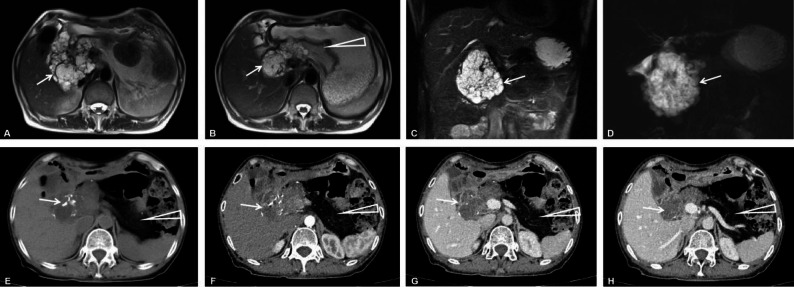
Imaging features. (A, B) Axial magnetic resonance images demonstrate a cystic-solid mass located at the hepatic hilum and pancreatic head, exhibiting a multicystic pattern with visible septations (↑). (C) Coronal magnetic resonance image showing a cystic-solid mass at the hepatic hilum and pancreatic head, also presenting a multicystic pattern with septations (↑). (D) MR cholangiopancreatography shows no visualization of the main pancreatic duct. (A) Plain CT scan reveals a mixed-density mass with internal calcifications. (B) Arterial phase CT shows mild enhancement of the lesion. (C, D) Venous phase delayed enhancement images reveal a multilocular cystic mass in the pancreatic head, composed of numerous small locules with lobulated contours, and central enhancing calcifications (↑). The pancreatic body and tail region is completely replaced by fatty tissue without identifiable pancreatic duct (△)
